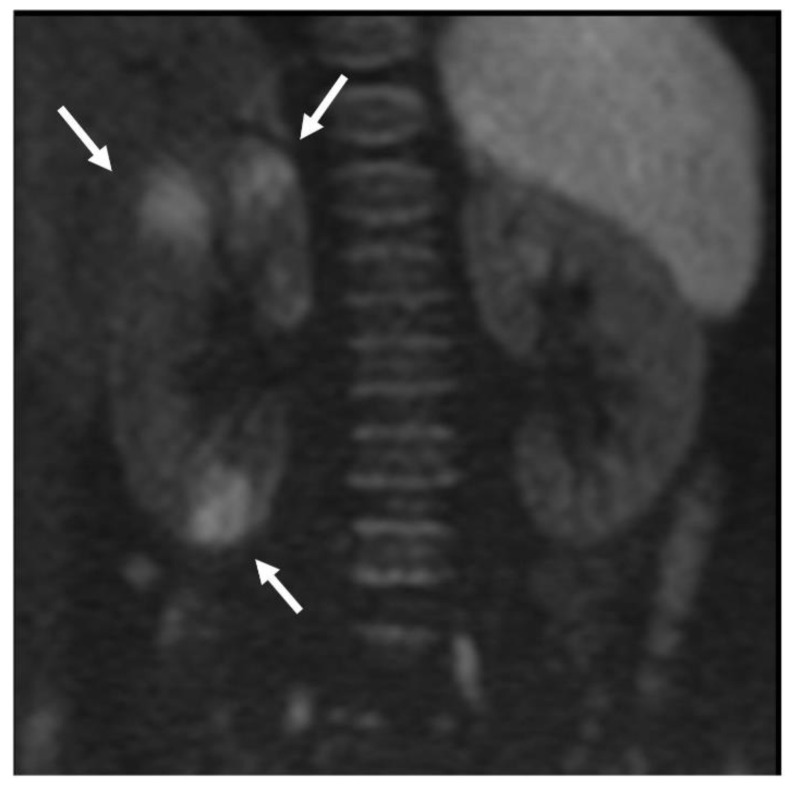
Patient with fUTI: signs of acute parenchymal involvement (multiple focal renal lesions) detected by DWI (*b*-value diffusion = 1000).
